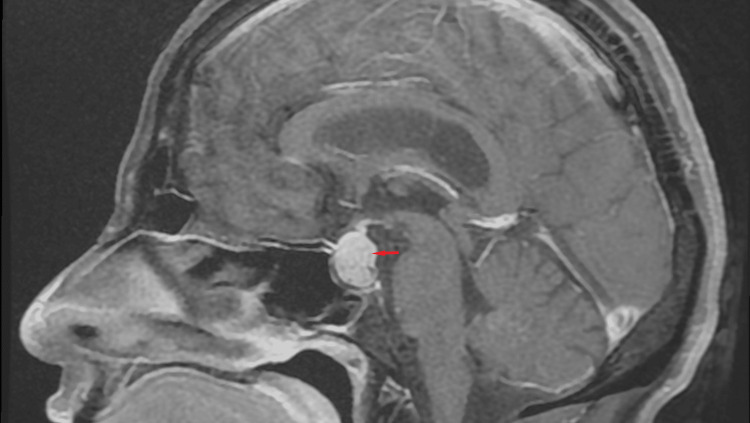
Pituitary sagittal T1W after gadolinium injection MRI image showing a pituitary macroadenoma of 16x16x12 mm diameter with parasellar extension and without compression of the optic chiasm
